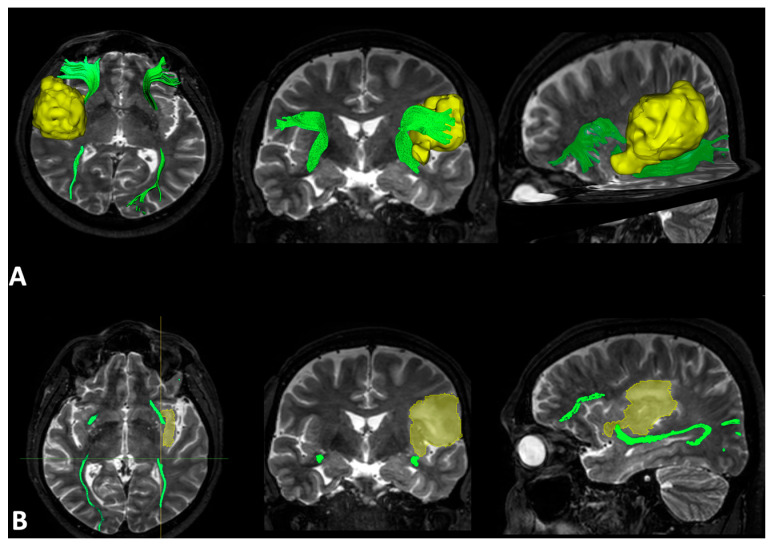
Left hemisphere temporo-insular low-grade glioma: (A) the qualitative analysis shows left IFOF (in green) downward displacement in axial and sagittal planes in 3D neuroradiological view of the tumor (in yellow). (B) T2-weighted sequences showing axial, coronal, and sagittal view of the tumor (in yellow) and the course of IFOF in the healthy and tumoral hemisphere (in green).
